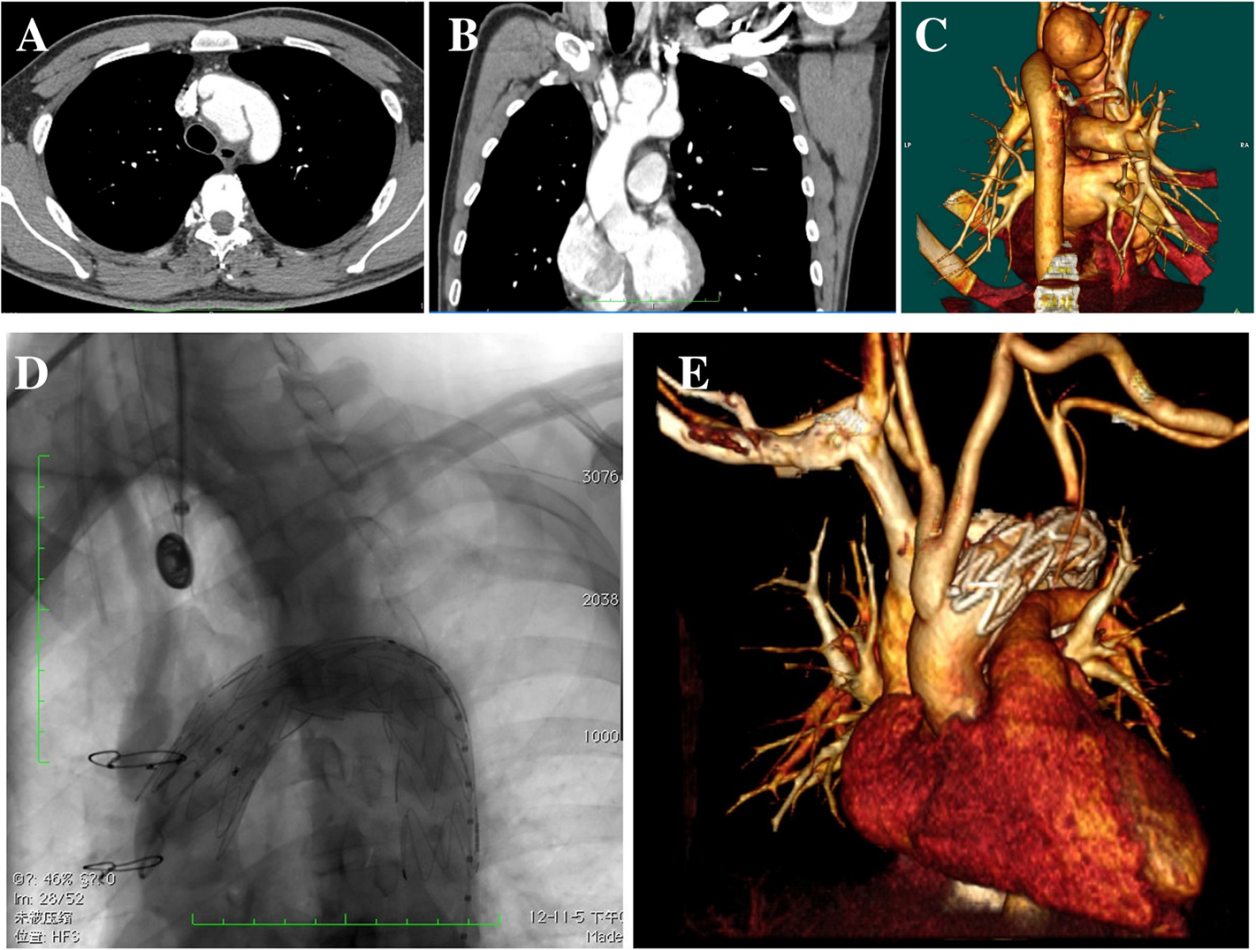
(a-c) Preoperative CT; (d) Intraoperative angiography; (e) CT angiography on the 7th postoperative day
